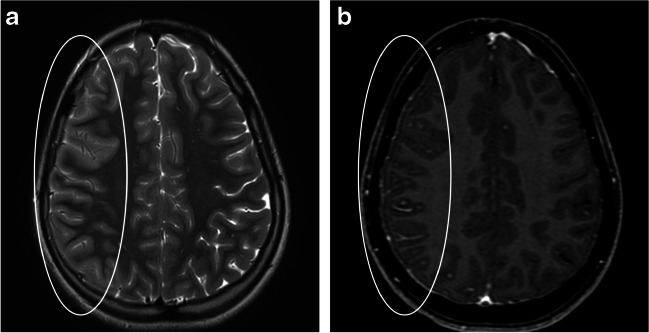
Cortical encephalitis in myelin oligodendrocyte glycoprotein antibody-associated disease. Magnetic resonance images of the brain obtained from a 14-year-old girl. a Axial T2-weighted image shows diffuse swelling of the right-sided cerebral cortex with sulcal effacement (*ellipse*). b Axial contrast-enhanced T1-weighted image shows asymmetric leptomeningeal enhancement along the involved region (*ellipse*)
